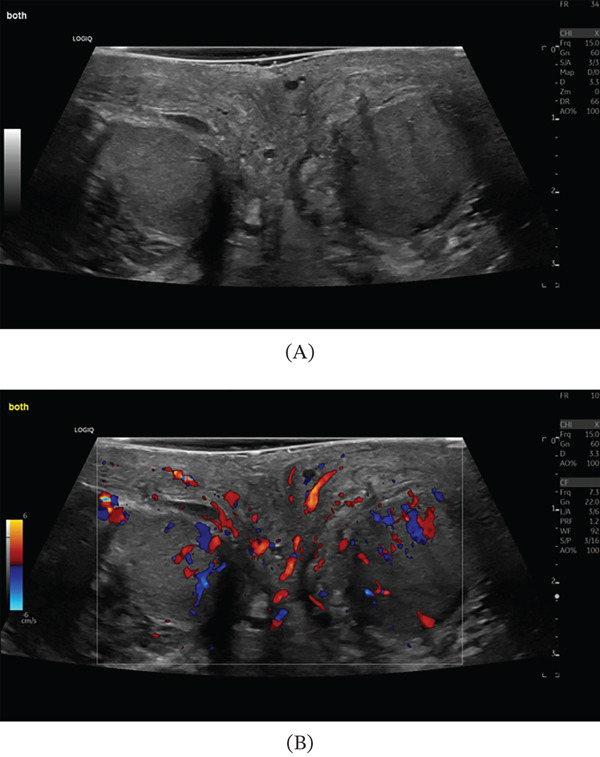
Immediate postoperative ultrasound (US) of both testes; (A) conventional US of both testes and (B) Doppler US of both testes demonstrating hyperaemia.
